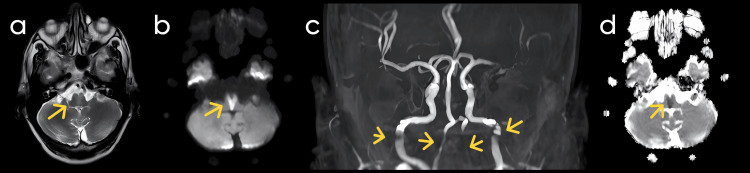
MRI Sequences of Case 1 (a) FLAIR (fluid-attenuated inversion recovery) imaging revealed hyperintensity involving the bilateral pyramids and central medulla. (b) DWI (diffusion-weighted imaging) demonstrated areas of diffusion restriction. (c) MR (magnetic resonance) angiogram showed flow restriction in both vertebral arteries and the basilar artery, with no evidence of dissection or occlusion. (d) ADC (apparent diffusion coefficient) mapping confirmed diffusion restriction in the affected region.
